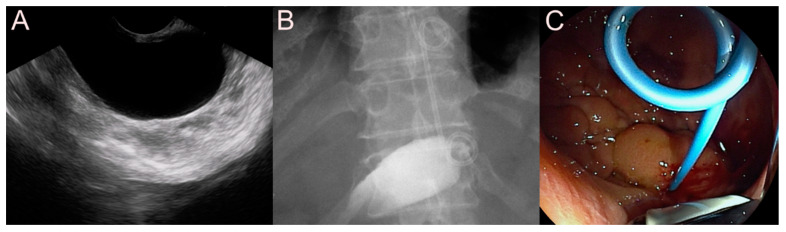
Endoscopic ultrasound (EUS)-guided pancreatic fluid collection (PFC) drainage using the 7 Fr double-pigtail plastic stent (DPPS) for a pseudocyst. (A) Endosonographic image showing a pseudocyst without sludge and heterogeneous debris. (B) Fluoroscopic image showing the DPPS placed between the stomach and the pseudocyst. (C) Endoscopic image showing the drainage of dark brown fluid materials and minimal sludge through the EUS-guided PFC drainage.
